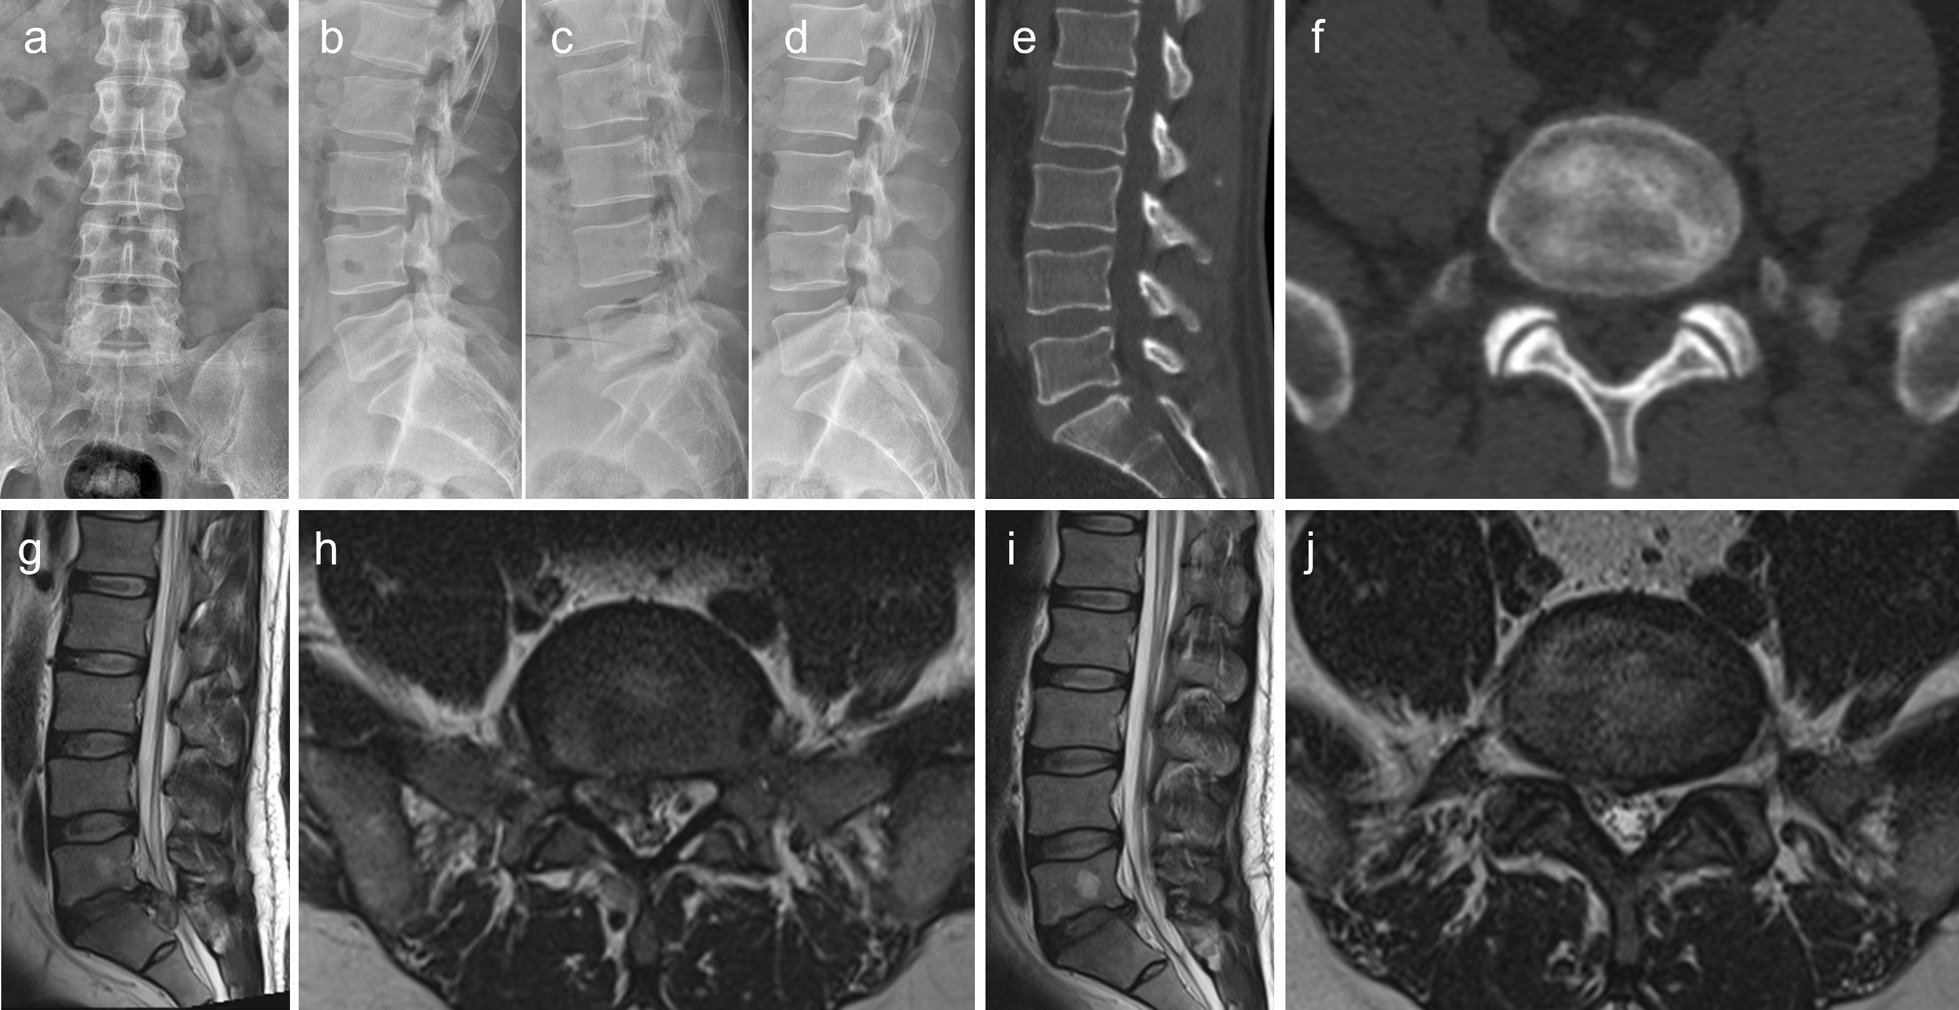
A 42-year-old man with highly downward-migrated disc herniation was treated with percutaneous endoscopic interlaminar discectomy. The patient mainly complained of low back pain with radiating pain in the right lower limb and cauda equina symptoms. The degree of prominence is very high grade. a, b Anterior and lateral lumbar radiographs; c, d Dynamic lumbar radiographs; e, f Computed tomography scan of the lower lumbar spine; g, h Magnetic resonance imaging (MRI) of the lumbar spine: Herniated disc at the L_5_-S_1_ segment, nucleus pulposus migration to the posterior edge of S_1_; i, j MRI revealing that the L_5_-S_1_ herniated disc and the free nucleus pulposus had been removed
